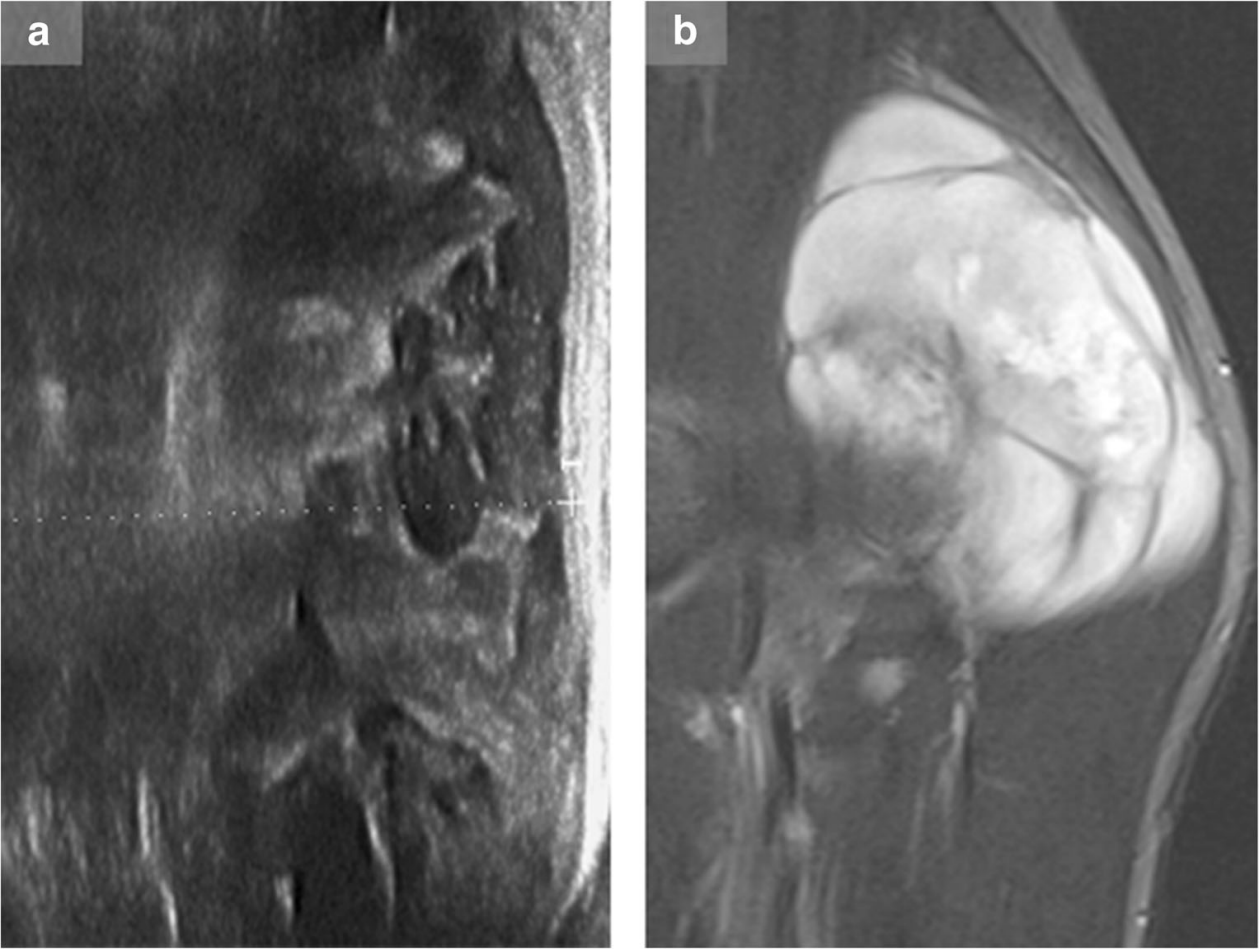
Tremendous growth of a lump-like mass, infiltrative aspects, changed vascularity, and random heterogeneity must raise attention to malignancy. In this figure, ultrasound (a) and corresponding MRI images (b; short TI inversion recovery (STIR)–weighted sequence of the same tumor, sagittal plane) of a malignant peripheral nerve sheath tumor in NF1 are shown
